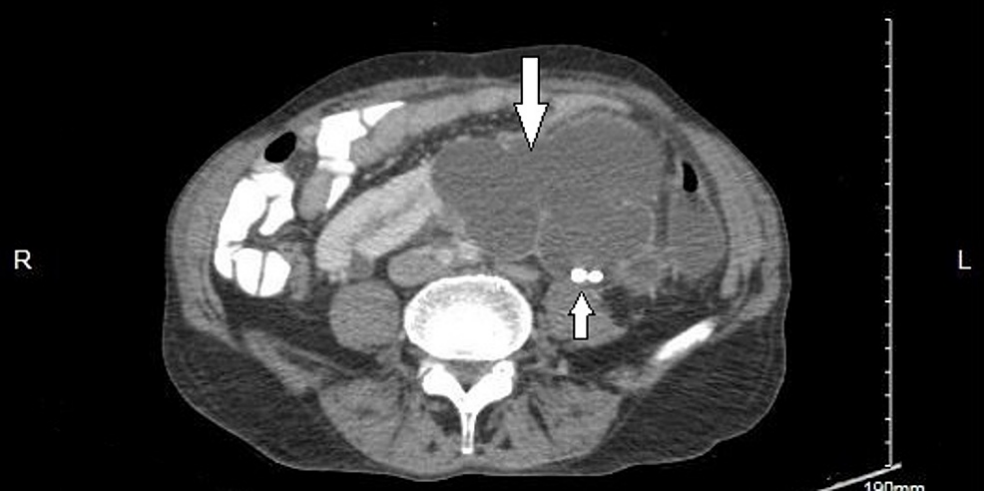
Axial CT view showing left kidney with severe hydronephrosis and multiple stones. CT: computed tomography.
